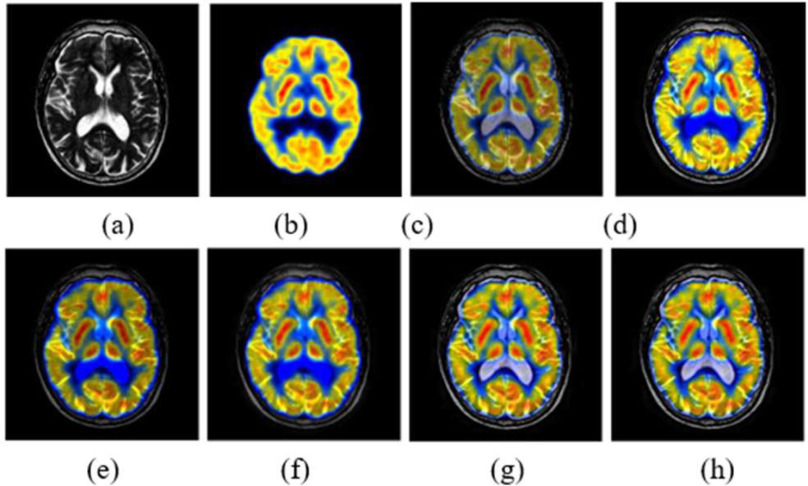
MRI-PET fusion outcomes (a) MR input, (b) PET input (c) MCA-CS [14], (d) LTEM [15], (e) DB-CNN [17], (f) GF-SDL [18], (g) LDNSD [19], (h) Proposed fused outcome.
